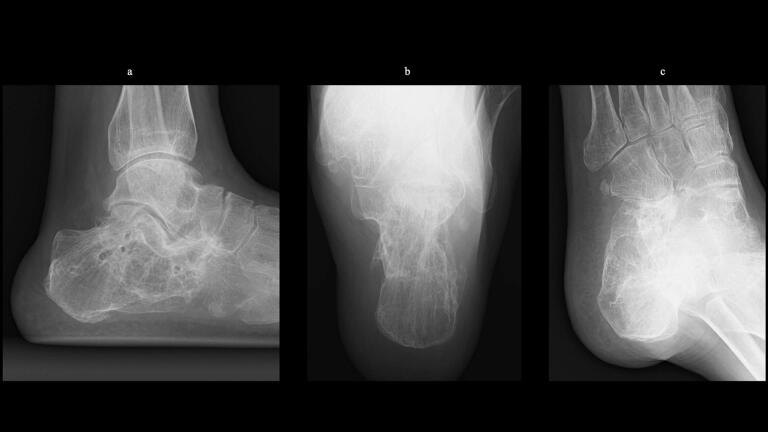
Final X-ray 16 months after surgery. After removal of the plate without correction loss. a: lateral view of the calcaneus. b: axial view of the calcaneus. c: Oblique view of the foot.
